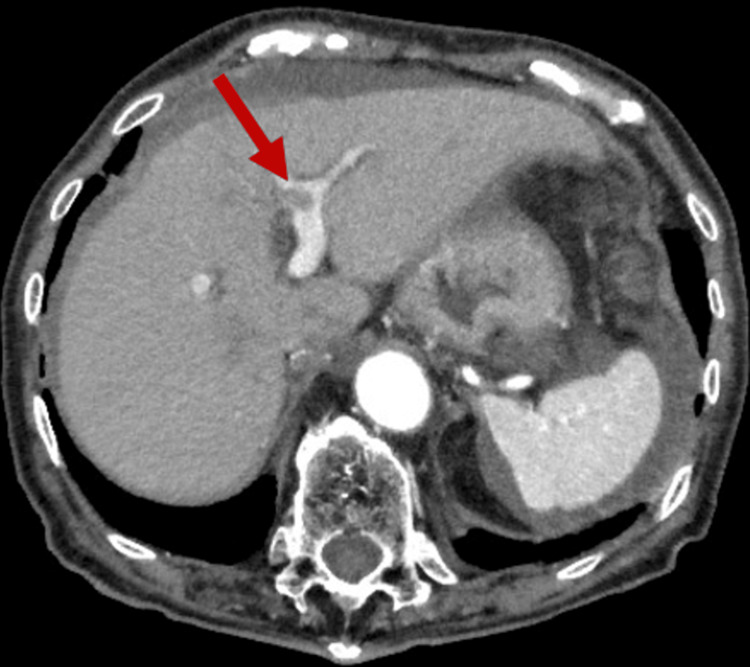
CT revealing ascites around the liver and a tumor thrombus in the portal vein (red arrow) CT: computed tomography
